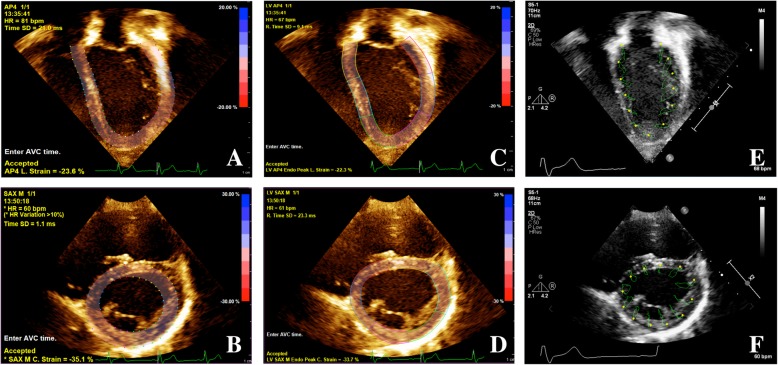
Speckle Tracking Packages Used for Assessment. Screenshots from the various speckle tracking packages. a) QLAB 10.5 (AP4 view), b) QLAB 10.5 (SAXM view), c) QLAB 10.8 (AP4 view), d) QLAB 10.8 (SAXM view), e) TomTec (AP4 view), f) TomTec (SAXM view). AP4 (Apical four chamber view), SAXM (Short axis mid-ventricular papillary muscle)
